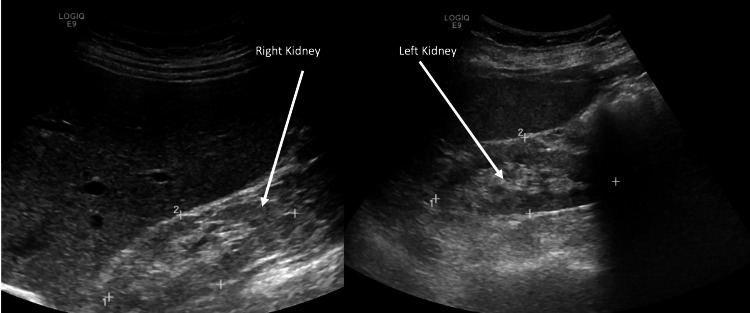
Renal ultrasound with increased echogenicity of renal parenchyma
